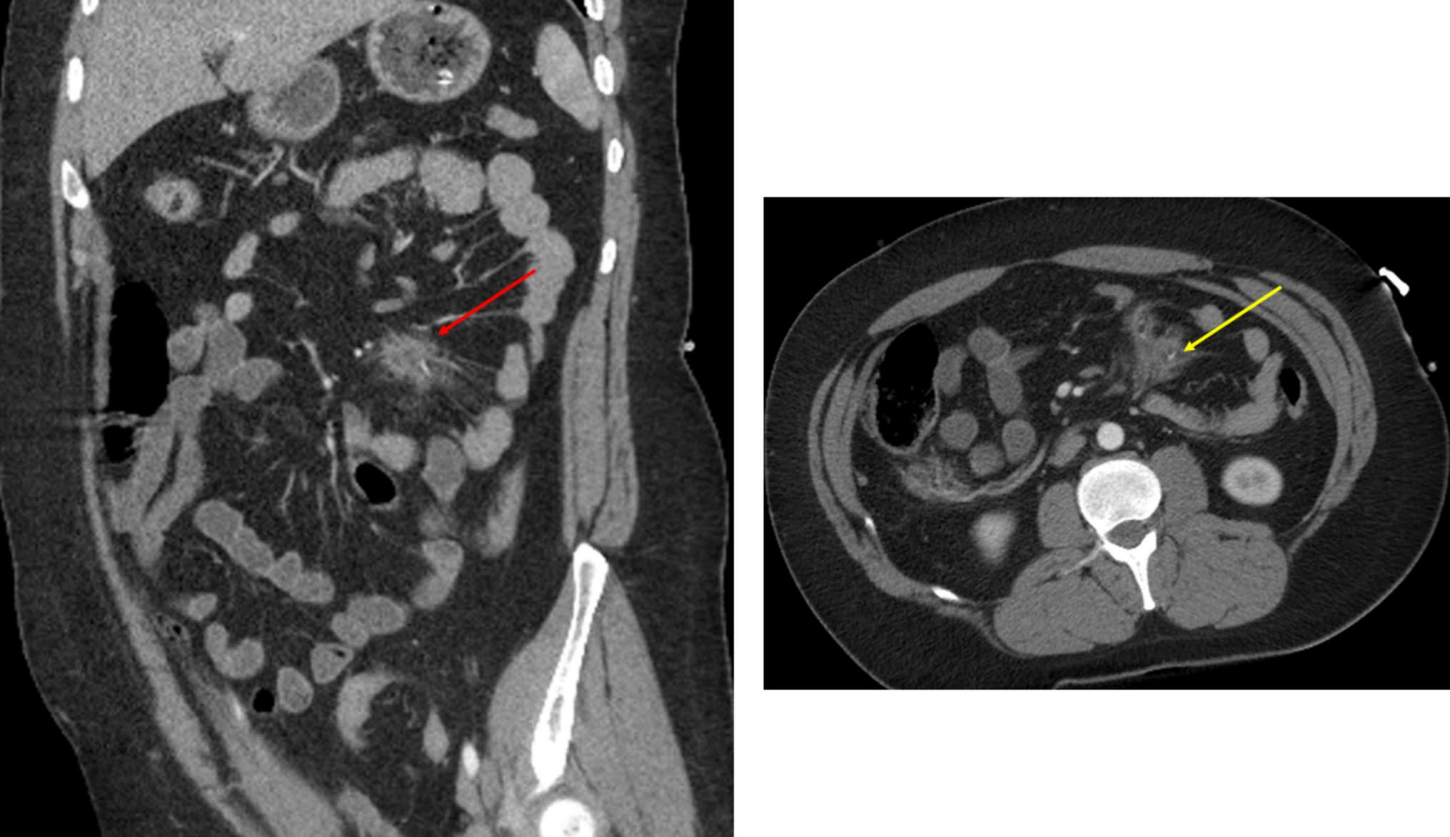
(Left) Coronal CT of the abdomen demonstrates a small mesenteric contusion (red arrow). (Right) Axial CT shows the mesenteric contusion with a small focus of active extravasation (yellow arrow). This patient had no bowel injury upon exploratory laparotomy
